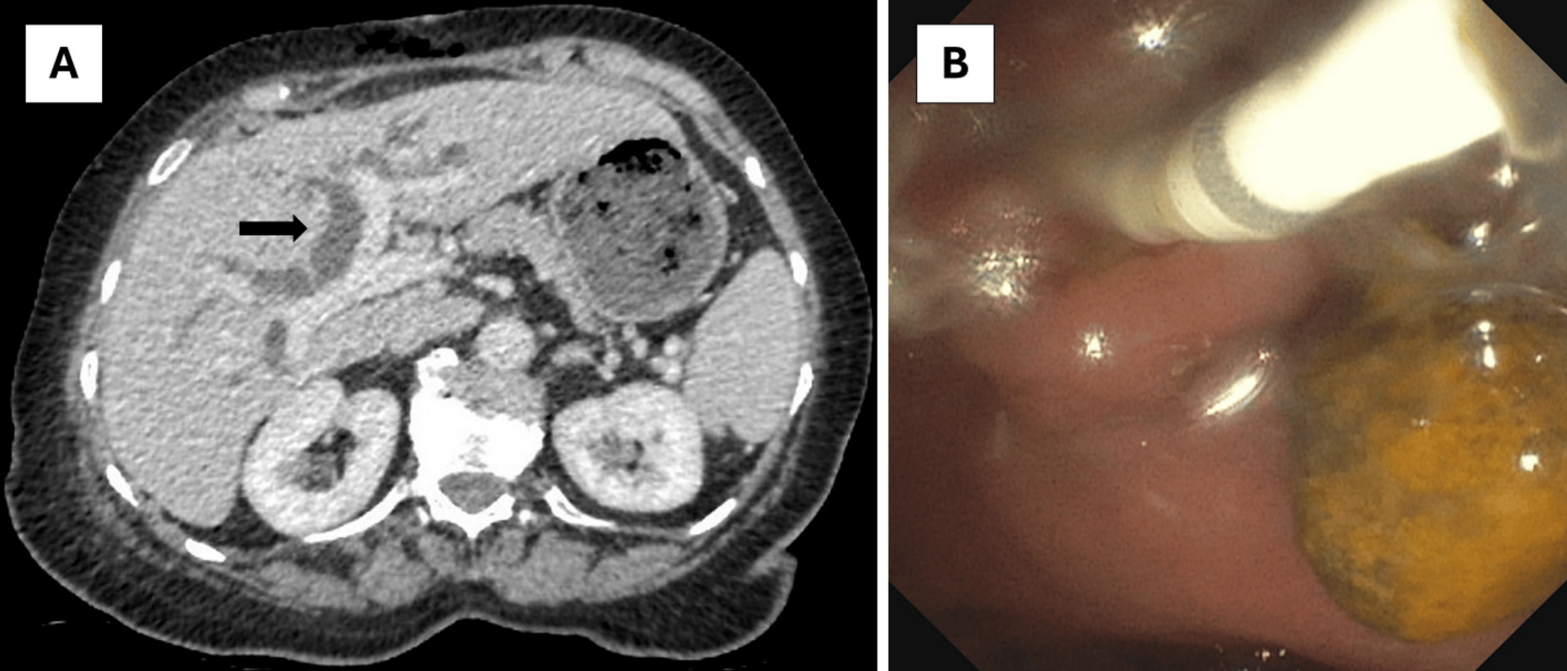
Postoperative CT and ERCP Panel A: Computed tomography (CT) with intravenous contrast showing marked dilatation of the intrahepatic bile ducts (arrow). Panel B: View during endoscopic retrograde cholangiopancreatography (ERCP) after sphincterotomy and removal of the obstructing gallstone.
